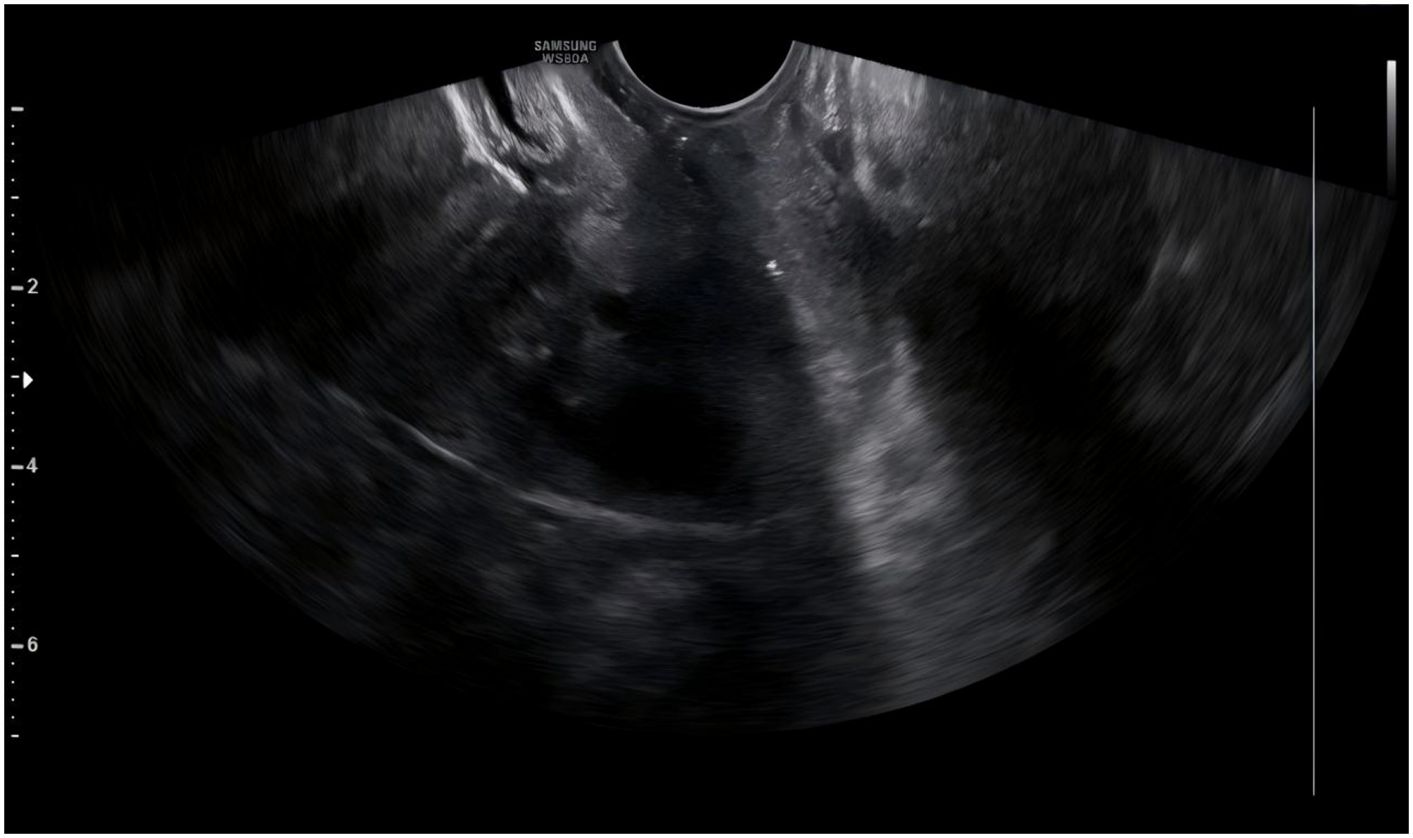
The patient's vaginal ultrasound indicates LNG - IUS displacement into the vagina.
